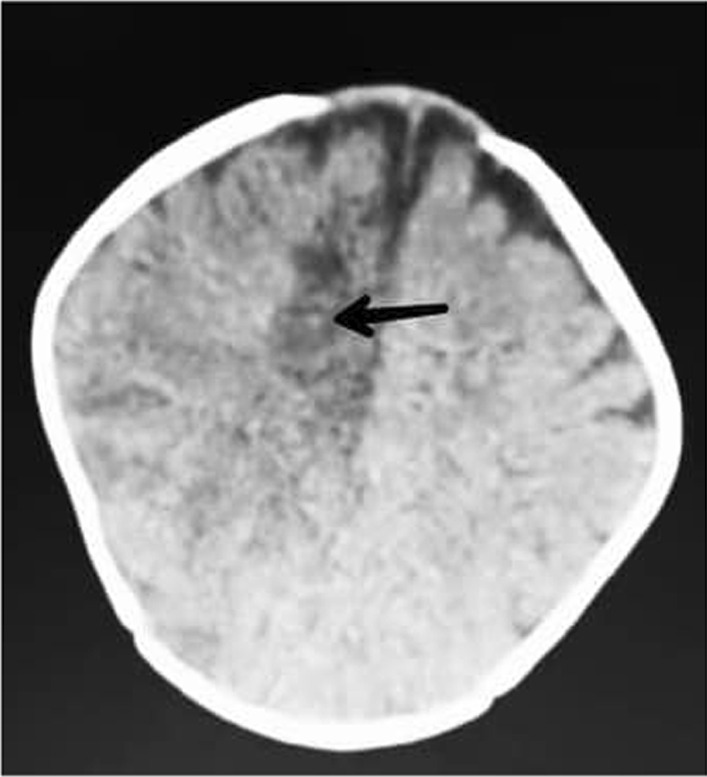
Brain computed tomography scan showing right parietal lobe ischemia (black arrow)
